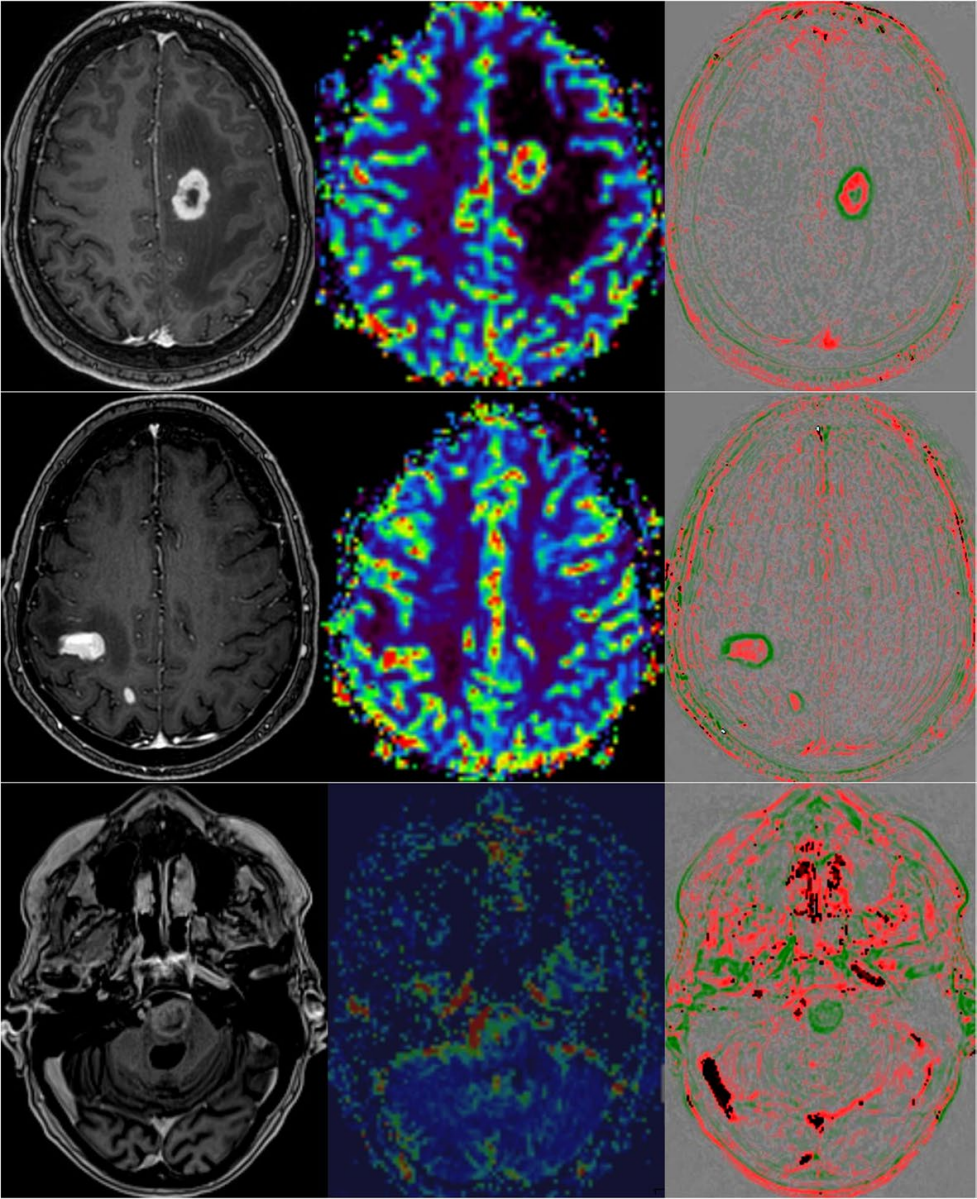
Examples of initial diagnosis (top: glioblastoma; middle: CNS lymphoma; bottom: brain metastasis of a small cell lung carcinoma). Left: contrast enhanced T1-weighted images; middle rCBV map of MR perfusion; right: rapid wash-out map
